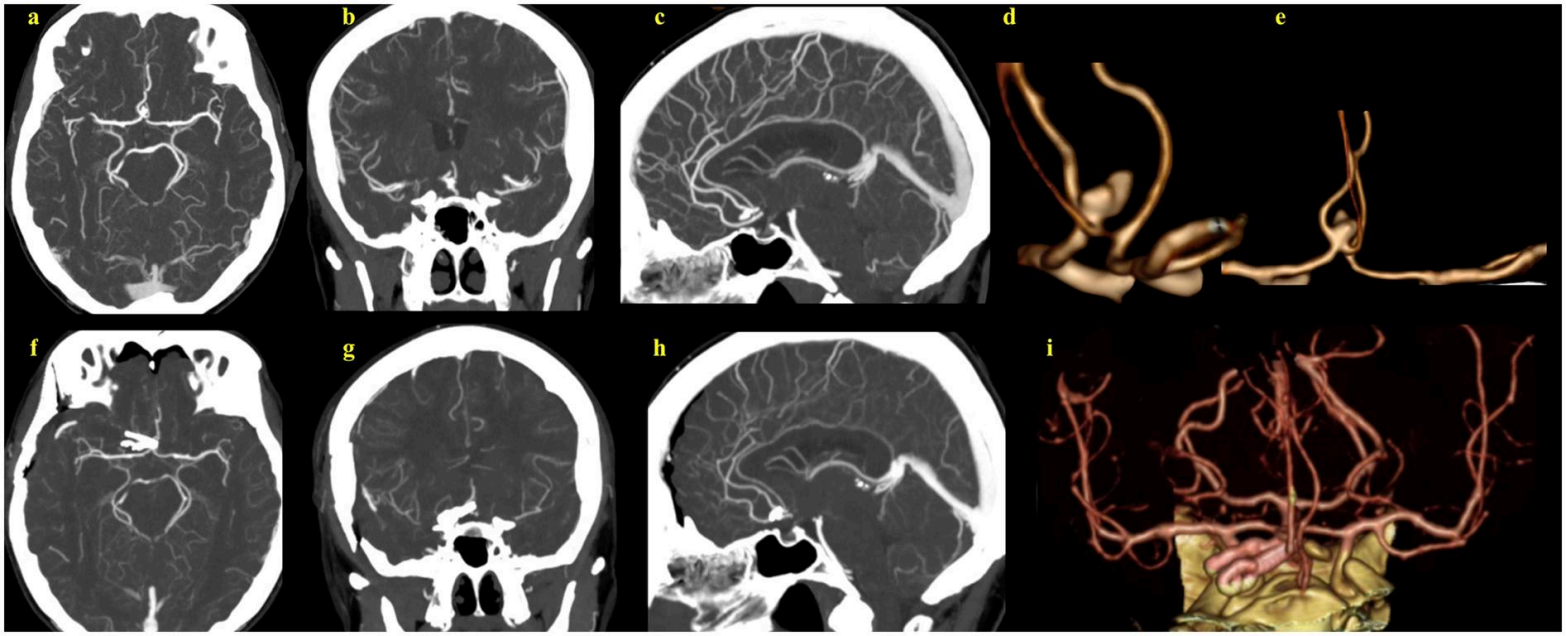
(a–c) Preoperative CT angiograms of the complex multilobular anterior communicating artery aneurysm. (d,e) 2D reconstructions of the preoperative CT angiographic images, providing enhanced aneurysm visualization and its relation to adjacent vascular structures. (f–h) Postoperative CT angiograms show successful aneurysm clipping. (i) 2D reconstruction of the postoperative images.
